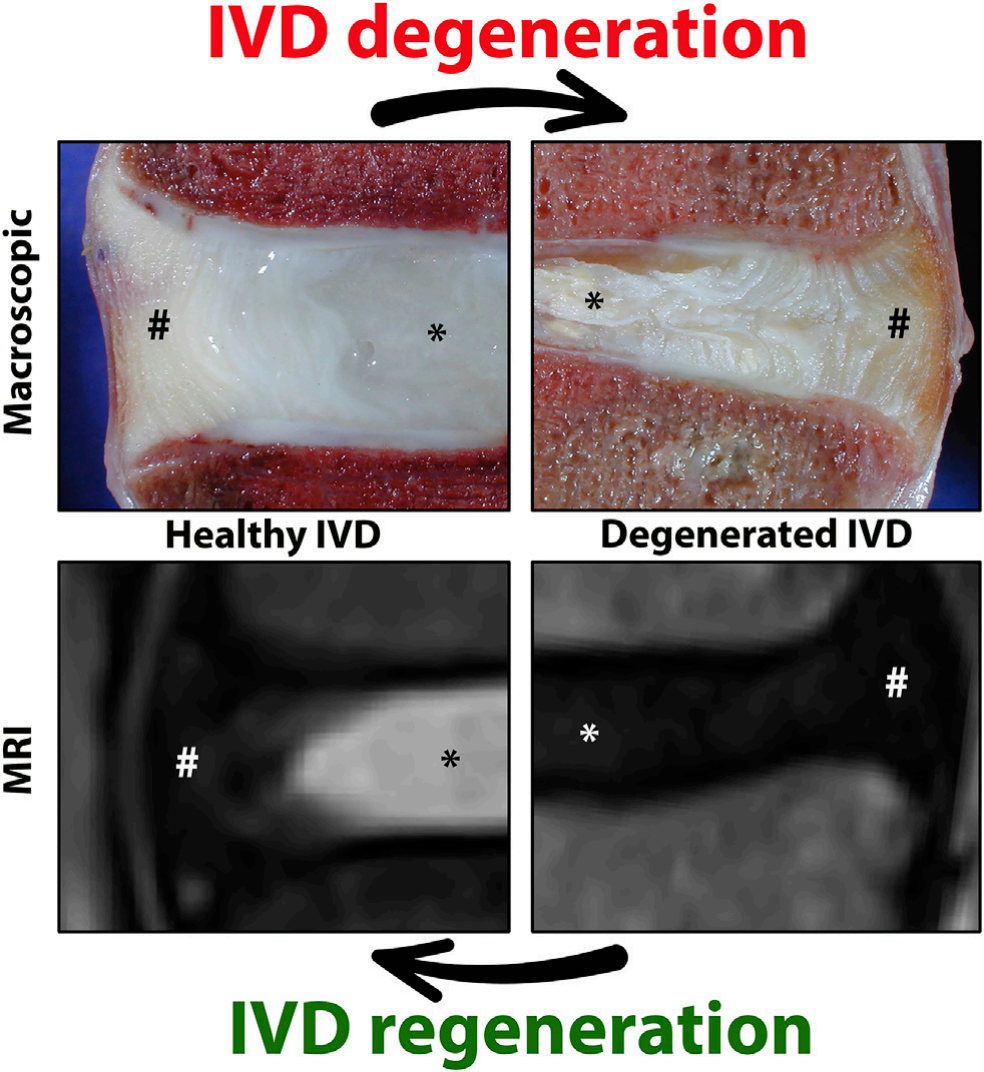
Representation of the healthy and degenerated human intervertebral disc (IVD) at macroscopic and magnetic resonance imaging typically used to visualize the IVD. Human healthy and degenerated L4-L5 IVDs are employed, a location commonly affected in chronic low back pain. The core of the IVD [nucleus pulposus, NP (*)] is constrained by the two endplates and the annulus fibrosus [AF(#)]. A healthy IVD has a gel-like NP with high water and glycosaminoglycan content (white on the T2 weighted sequence). IVD degeneration starts with loss of glycosaminoglycan and water within the NP (black on T2w sequence). Images by courtesy of Prof. Joachim Wilke.
